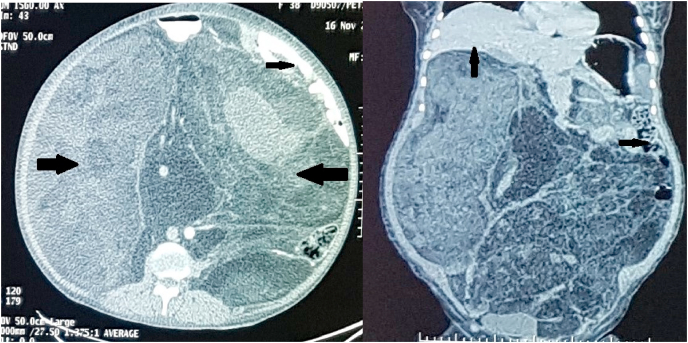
Cross-sectional and coronal CT images of the abdomen: large heterogeneously enhancing mass with a predominantly fat component occupying almost the entire abdominopelvic cavity (large arrows) with cranial displacement of the liver and small bowel in left hypochondrium (small arrows).
